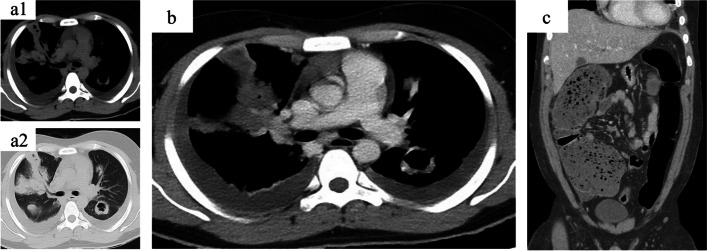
Contrast-enhanced computed tomography on day 6 of hospitalization. Multiple infiltrative shadows with associated cavities were observed (1-a1 & 1-a2). No pulmonary artery aneurysm was detected (1-b). The abdominal scan revealed a dilated ascending colon (1-c)
